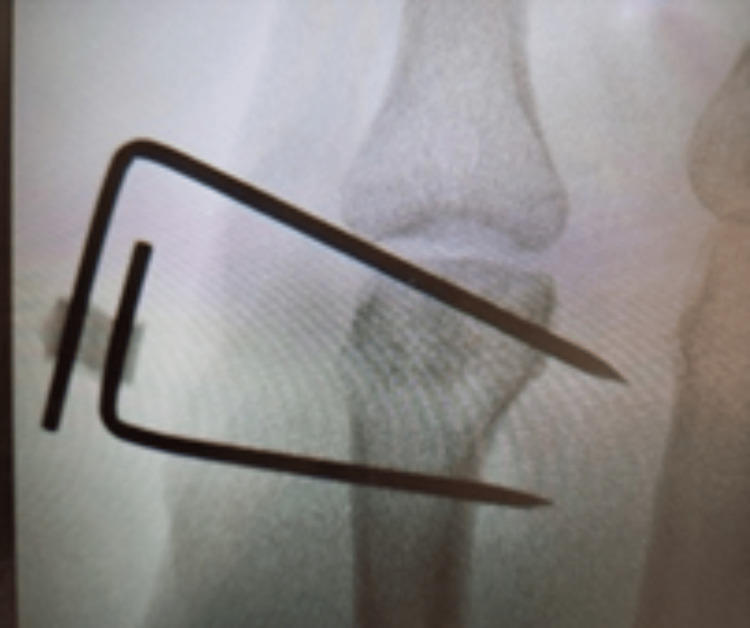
An intra-operative radiograph detailing the fixation device.
